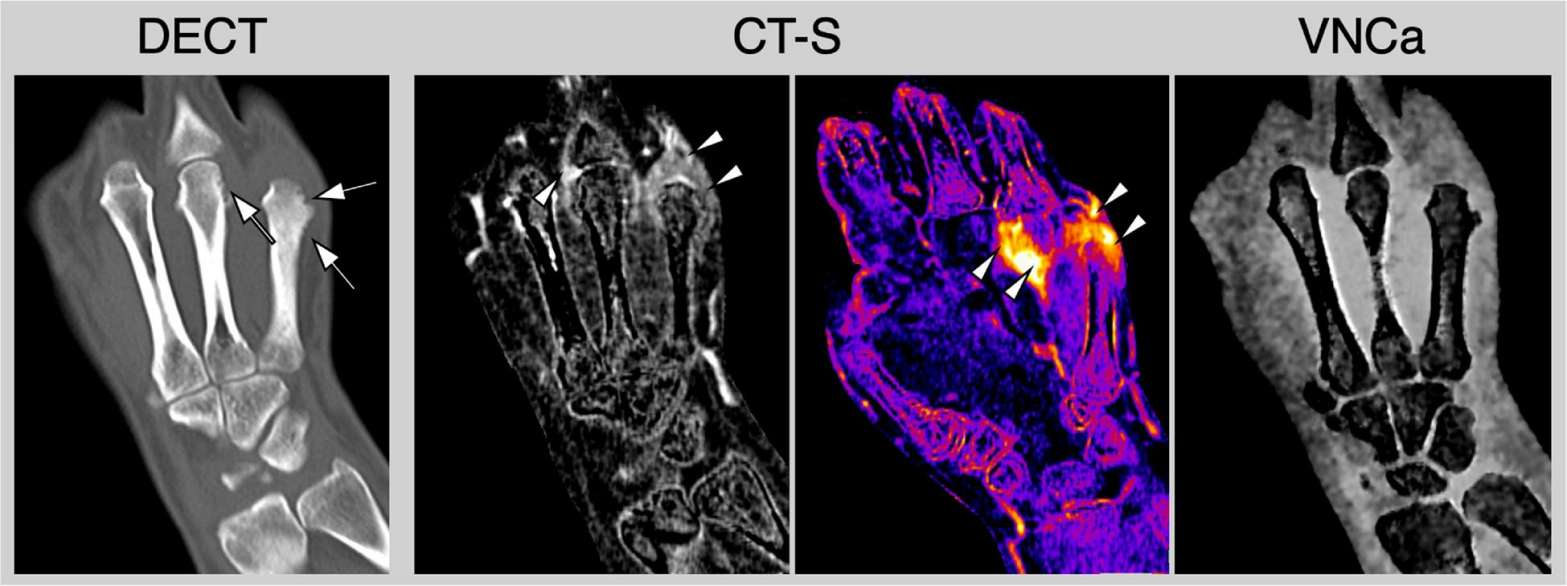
Multiparametric computed tomography in a patient with psoriatic arthritis. Conventional DECT imaging demonstrates both erosive changes and the presence of new bone formation (white arrows). The CT-S in greyscale and color-mapping techniques identifies active synovitis in the fourth and fifth metacarpophalangeal joints (white arrowheads). Additionally, VNCa does not show evidence of bone marrow edema in this patient who is diagnosed with active and erosive psoriatic arthritis
